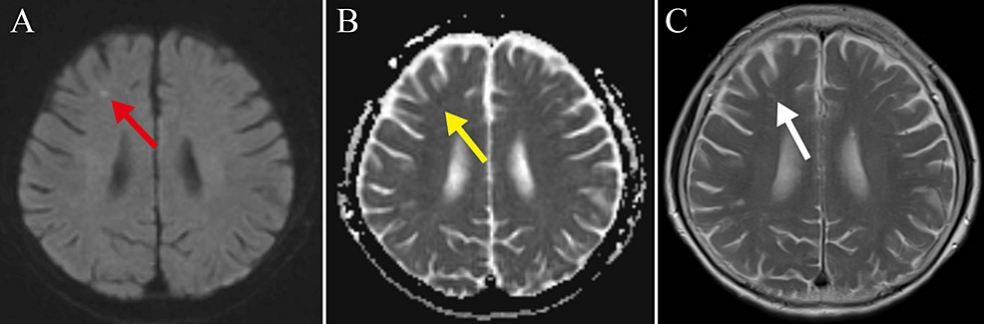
Cranial magnetic resonance imaging images two weeks after discharge. (A) Diffusion-weighted imaging shows a high signal in the right frontal lobe (red arrow). (B) Apparent diffusion coefficient map shows a low signal in the right frontal lobe (yellow arrow). (C) T2-weighted imaging shows a high signal in the right frontal lobe (white arrow).
